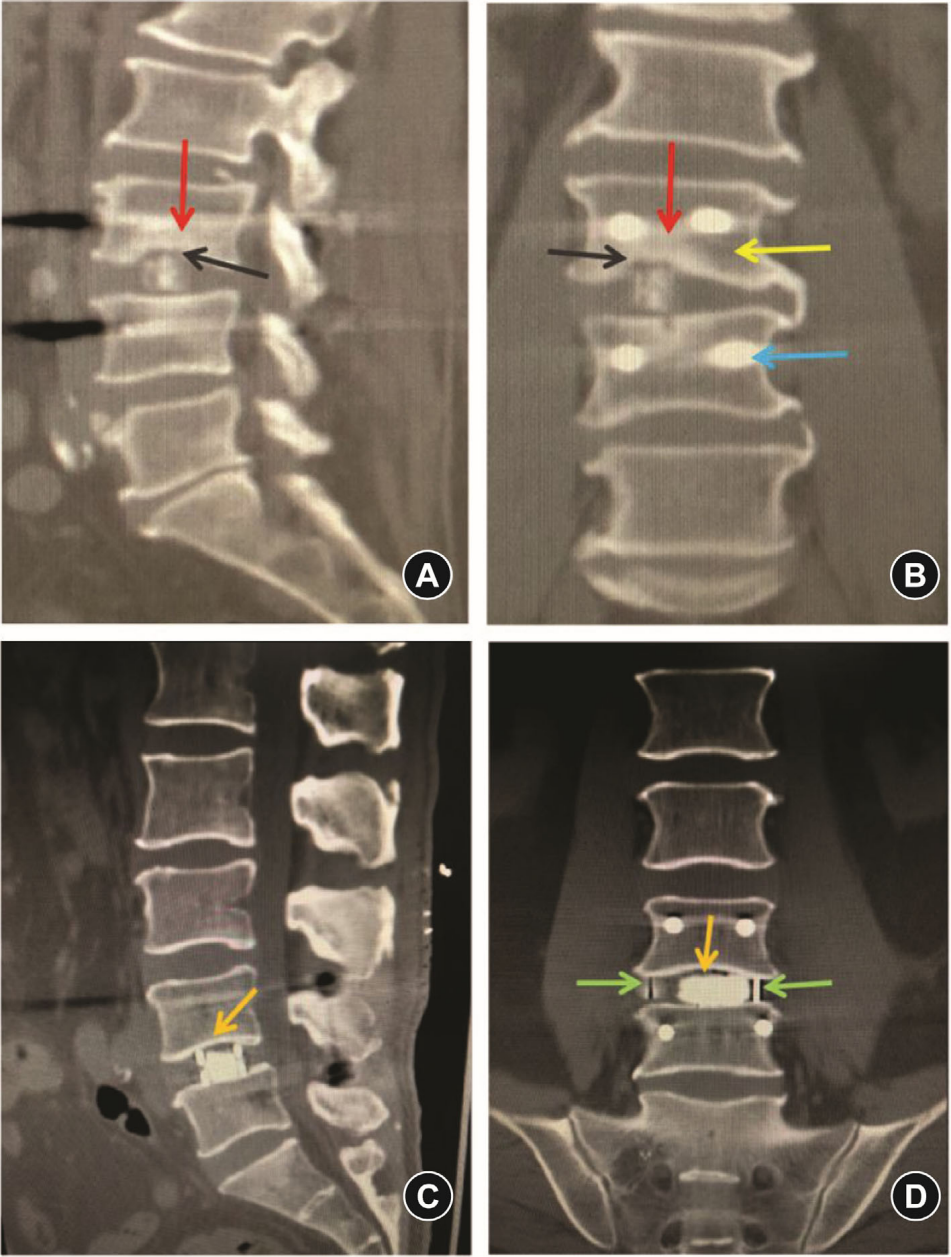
Computed tomography (CT) images after oblique lumbar interbody fusion (OLIF) and minimally invasive transforaminal lumbar interbody fusion (MI‐TLIF). (A) Sagittal CT image after MI‐TLIF. (A) Compression fracture occurred in the middle and lower part of the L3 vertebral body, which showed a marked increase in cancellous bone density in the vertebral body (red arrow). The lower end plate of L3 vertebral body collapsed (black arrow). The cage protrudes into the L3 vertebral body. (B) Anteroposterior image after MI‐TLIF. The cage was biased to the right of the vertebral body. There was a compression fracture in the middle and lower part of the right side of the L3 vertebral body, and the cancellous bone density on the right side of the vertebral body is significantly increased (red arrow). The bone density of the left vertebral body is normal (yellow arrow). The lower end plate of L3 vertebral body collapsed (black arrow). The cage protrudes into the L3 vertebral body. The cross section of the pedicle screw is shown by the blue arrow. (C) Sagittal CT image after OLIF. The lower end plate of L4 vertebral body and the upper end plate of L5 vertebral body were intact (orange arrows). There was no abnormal cancellous bone density in L4 and L5 vertebrae. (D) Anteroposterior image after OLIF. The markers on the left and right sides of the cage shown by green arrows, and the width of the cage is the same as the width of the vertebral body. The lower end plate of L4 vertebral body and the upper end plate of L5 vertebral body are intact (orange arrows). There was no abnormal cancellous bone density in L4 and L5 vertebrae
